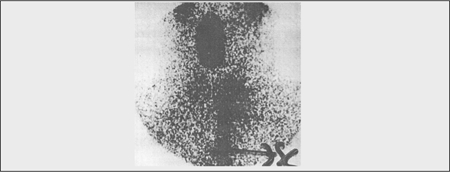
Low-dose I-131 scintigraphy after thyroidectomy
